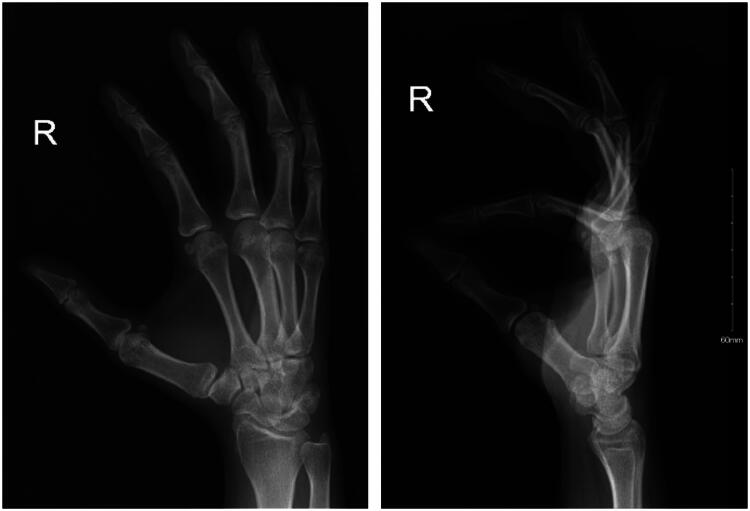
X-Ray images of the right hand in oblique, and lateral views.
